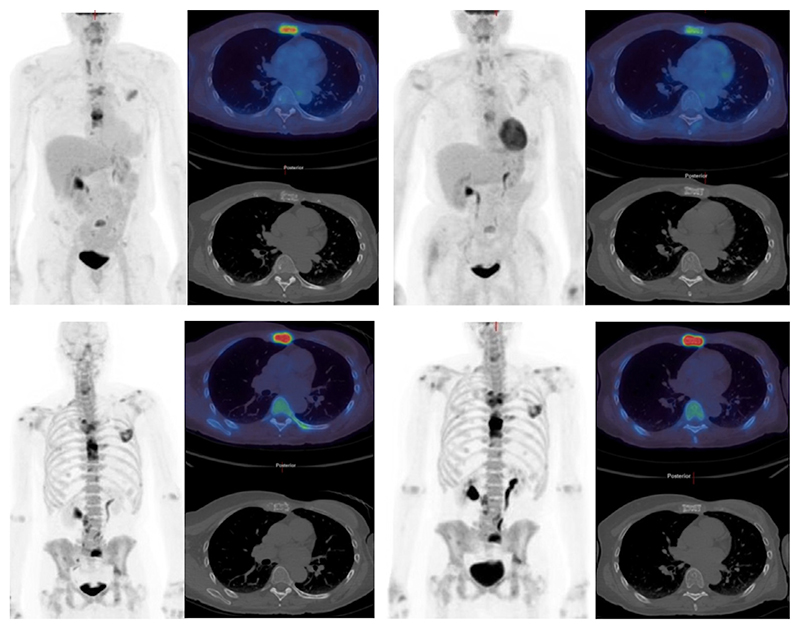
(a) ^18^F-FDG PET/CT maximum intensity projection image (left) and axial (right) images showing increased ^18^F-FDG uptake in a sternal metastasis with mixed lytic and sclerotic appearances on CT. After 8 weeks of endocrine treatment the corresponding images (right) show a reduction in uptake (SUVmax decreased from 11.5 to 5.3) confirming a partial metabolic response. (b) ^18^F-fluoride PET/CT images at the same time points showing an increase in activity in the sternal metastasis (SUVmax increased from 37.3 to 68.3) due to the flare phenomenon.
